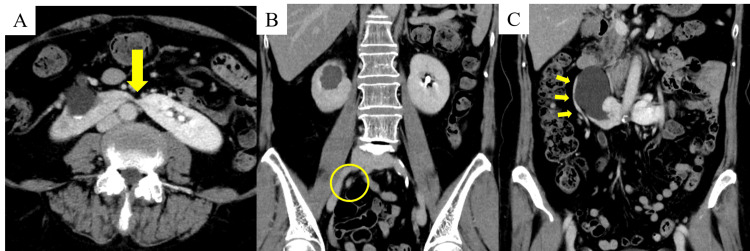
Contrast-enhanced computed tomography (CT) findings (A) Renal hilum of the horseshoe kidney (arrow). (B) Right hydronephrosis and a 5 × 5 mm solid tumor exhibiting contrast enhancement in the right lower ureter. (C) A single vascular branch originating from the distal right renal vein and extending toward the pelvis is observed around the right kidney (arrow).
